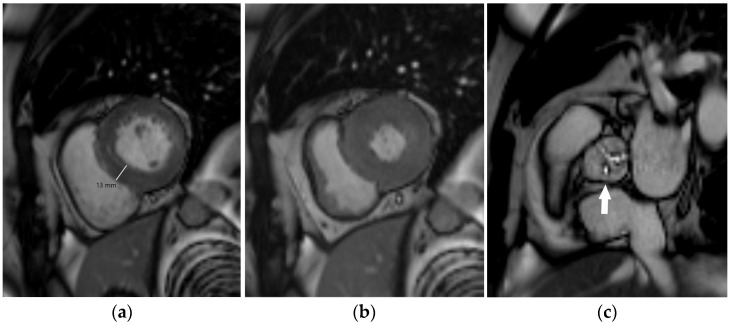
Cardiac MRI showing concentric left ventricular hypertrophy in an 80-year-old male patient with severe aortic stenosis. End-diastolic (a) and end-systolic (b) short-axis steady-state free precession (SSFP) images representing increased myocardial thickness (13 mm in end-diastole). SSFP image parallel to the aortic valve plane showing its impaired systolic opening (arrow, (c)).
